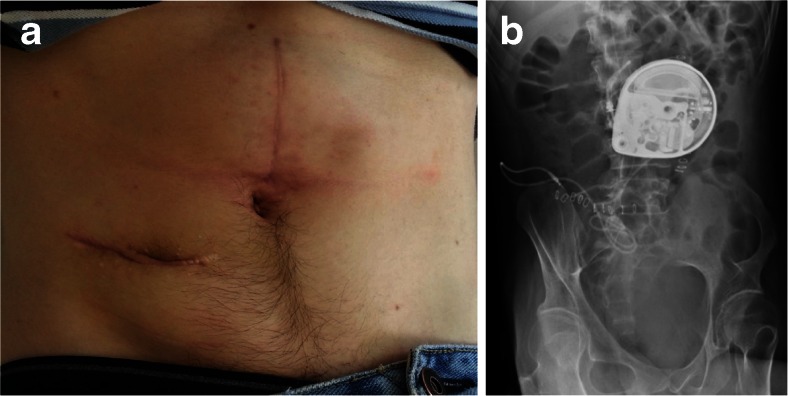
a Revised pump site 6 months postoperatively. Significantly less protrusion is seen with a subsequent decrease in tension on the overlying skin. b Erect abdominal radiograph depicting the re-sited baclofen pump in the posterior rectus fascial pouch. The catheter can be seen tracking into the right flank and onwards towards the spinal column
